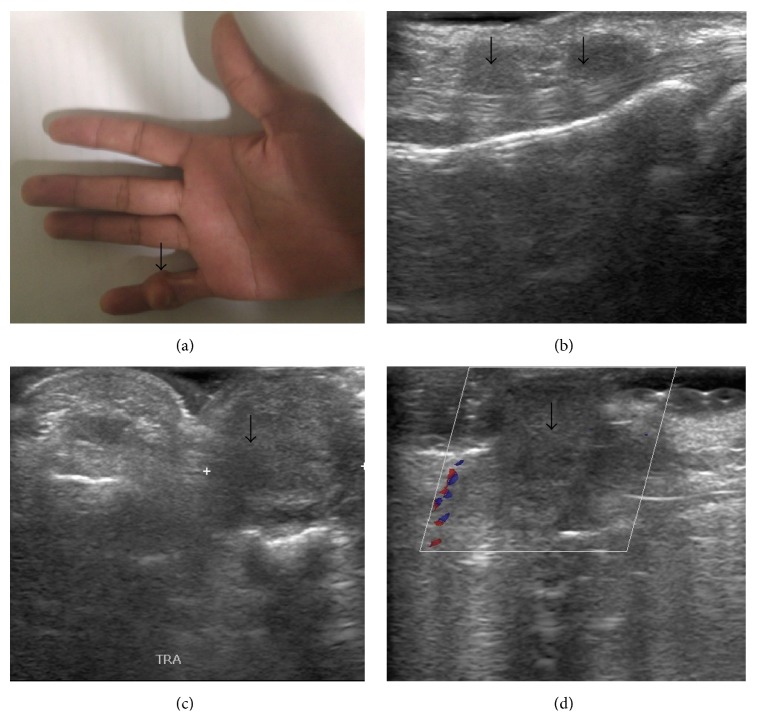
Giant cell tumor of tendon sheath. A 20-year-old female presented with swelling in right 5th finger over middle phalanx ((a), arrow). Ultrasonography and Doppler show multiple well-defined, hypoechoic, hypovascular lesions along the flexor tendons ((b)–(d), arrows).
